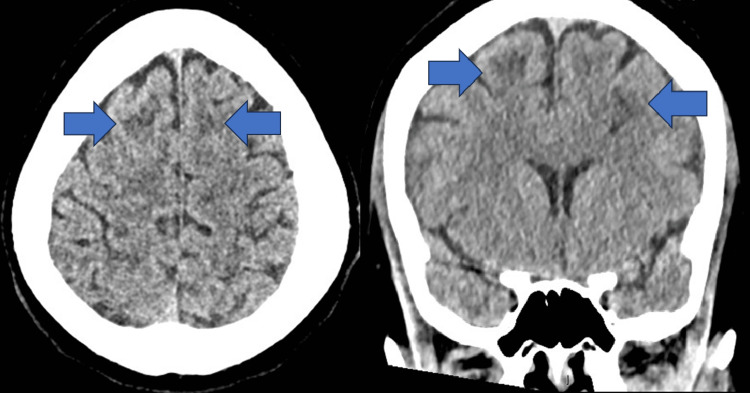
CT head without contrast showing focal hypodensities (blue arrows) apparent in both axial (left image) and coronal (right image) views
